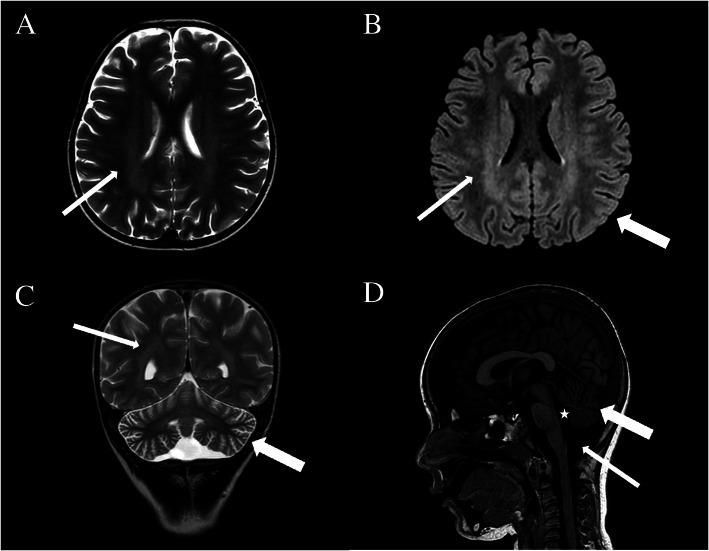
Brain magnetic resonance imaging (MRI) of patient #21. a Axial T2-weighted image showing mild hyperintensity in the periventricular deep white matter (thin arrow). b Axial fluid attenuated inversion recovery (FLAIR) scan demonstrating hyperintensity in the periventricular white matter, especially in the posterior regions (thin arrow), with preserved myelination in the subcortical white matter (thick arrow). c Coronal T2-weighted scan demonstrating mild hyperintensity in the posterior periventricular white matter (thin arrow) and moderate cerebellar atrophy (thick arrow). d Sagittal T1-weighted scan showing moderate cerebellar atrophy (thick arrow) with enlarged IV ventricle (star) and cisterna magna (thin arrow). The time from the first seizure to MRI was one month
